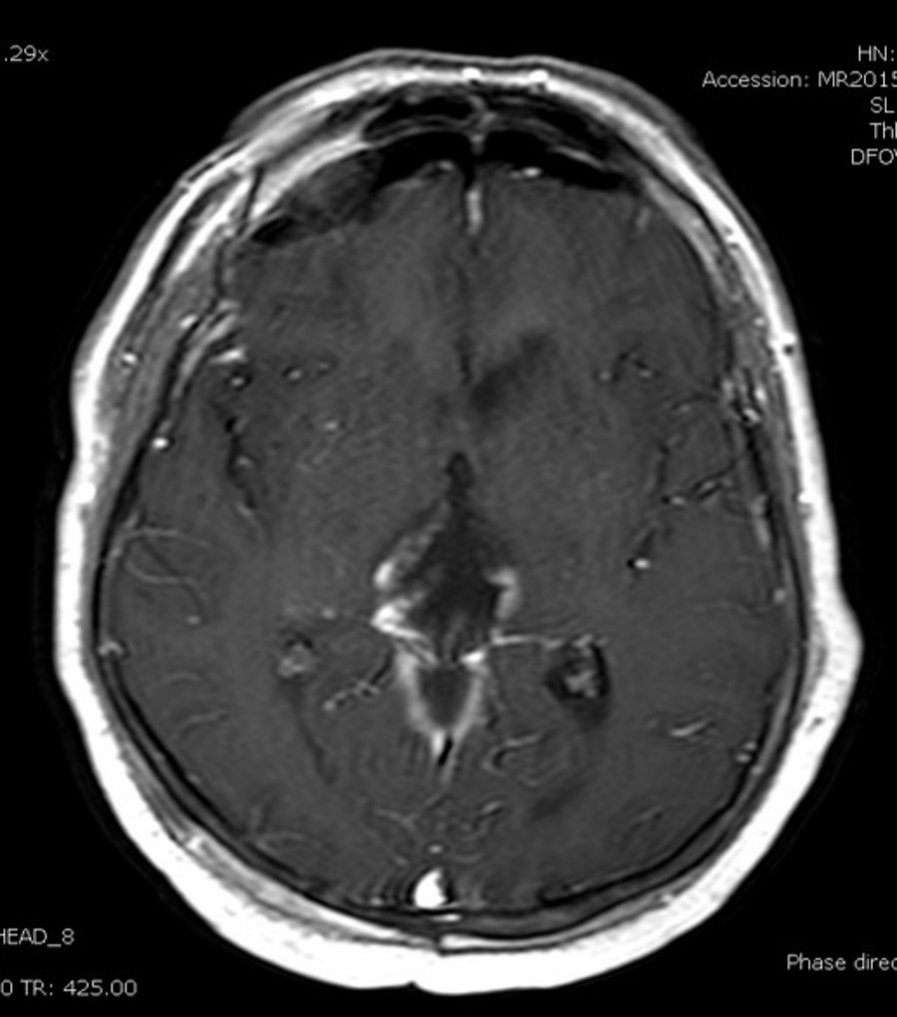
Postoperative MRI with contrast Postoperative T1 MRI with contrast showing close to gross total resection of the pineal glioma as compared to Figure 1.
